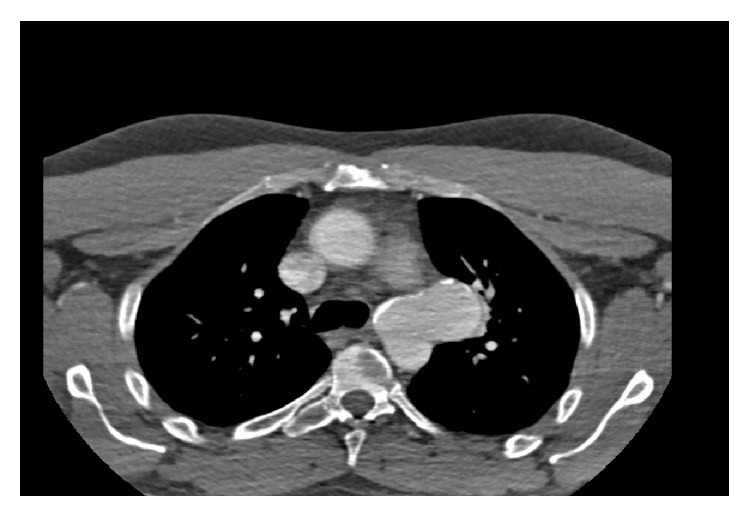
Transverse computed tomography imaging. Dense calcifications can be seen within the aneurysm wall.
